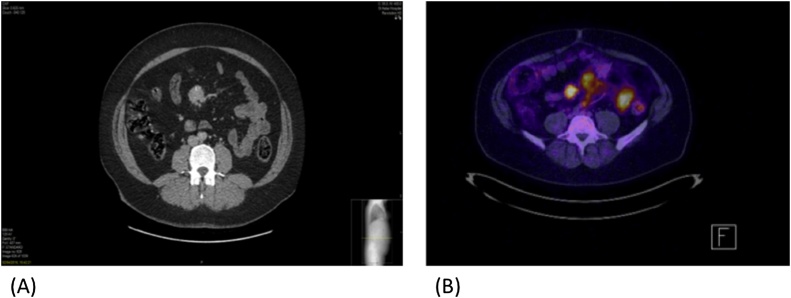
(A) Axial CT demonstrating a nodal mass within the mesentery anterior to the superior mesenteric vessels. (B) NM68 DOTATATE PET scan showing that avidity of the nodal mass and radiological appearances that are in keeping with a neuroendocrine tumour.
